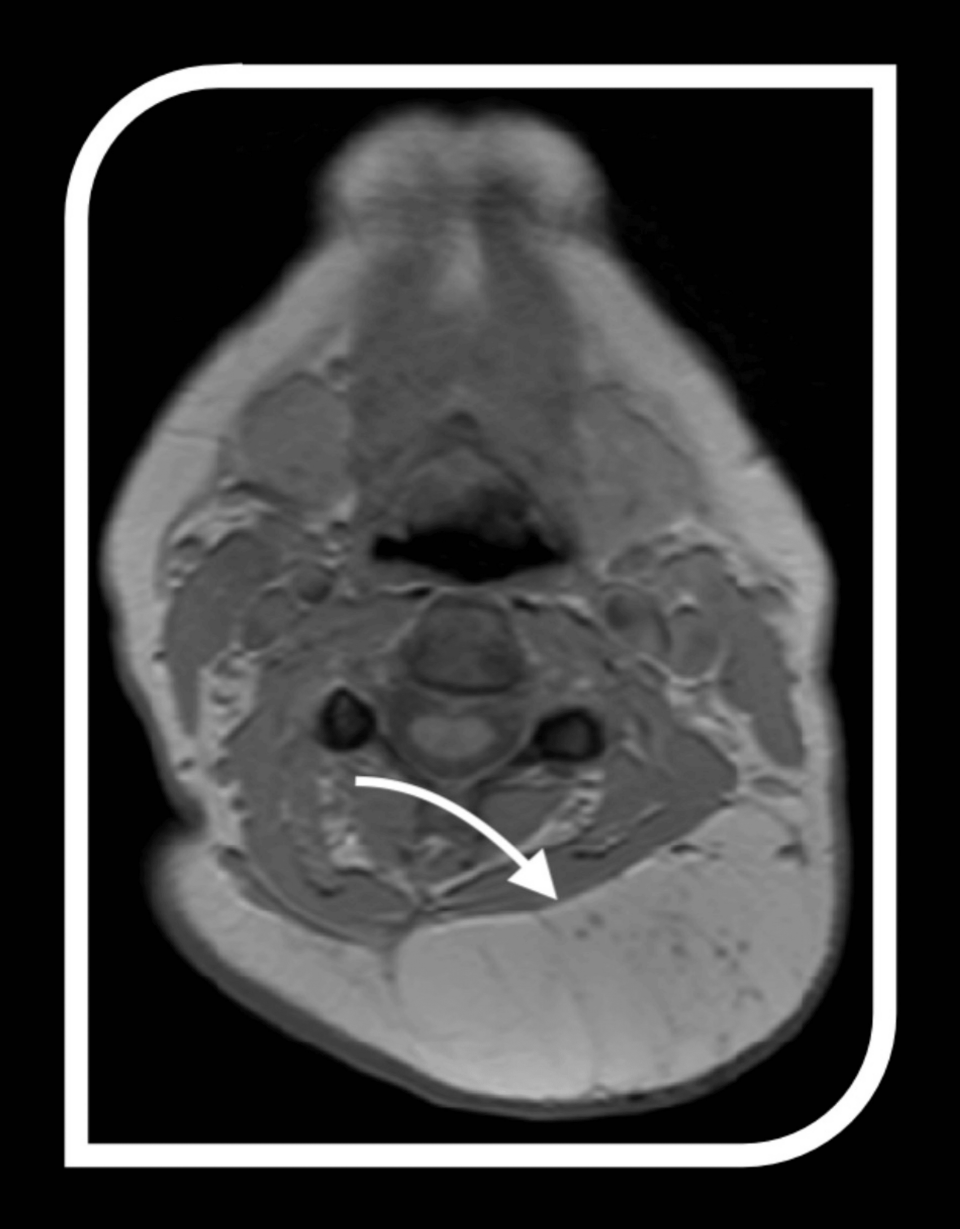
MRI imaging of atypical lipomatous tumours (ALTs) Representative features of ALTs on MRI employed in this study; the arrow indicates thick irregular septums and nodular contrast-enhanced areas.
